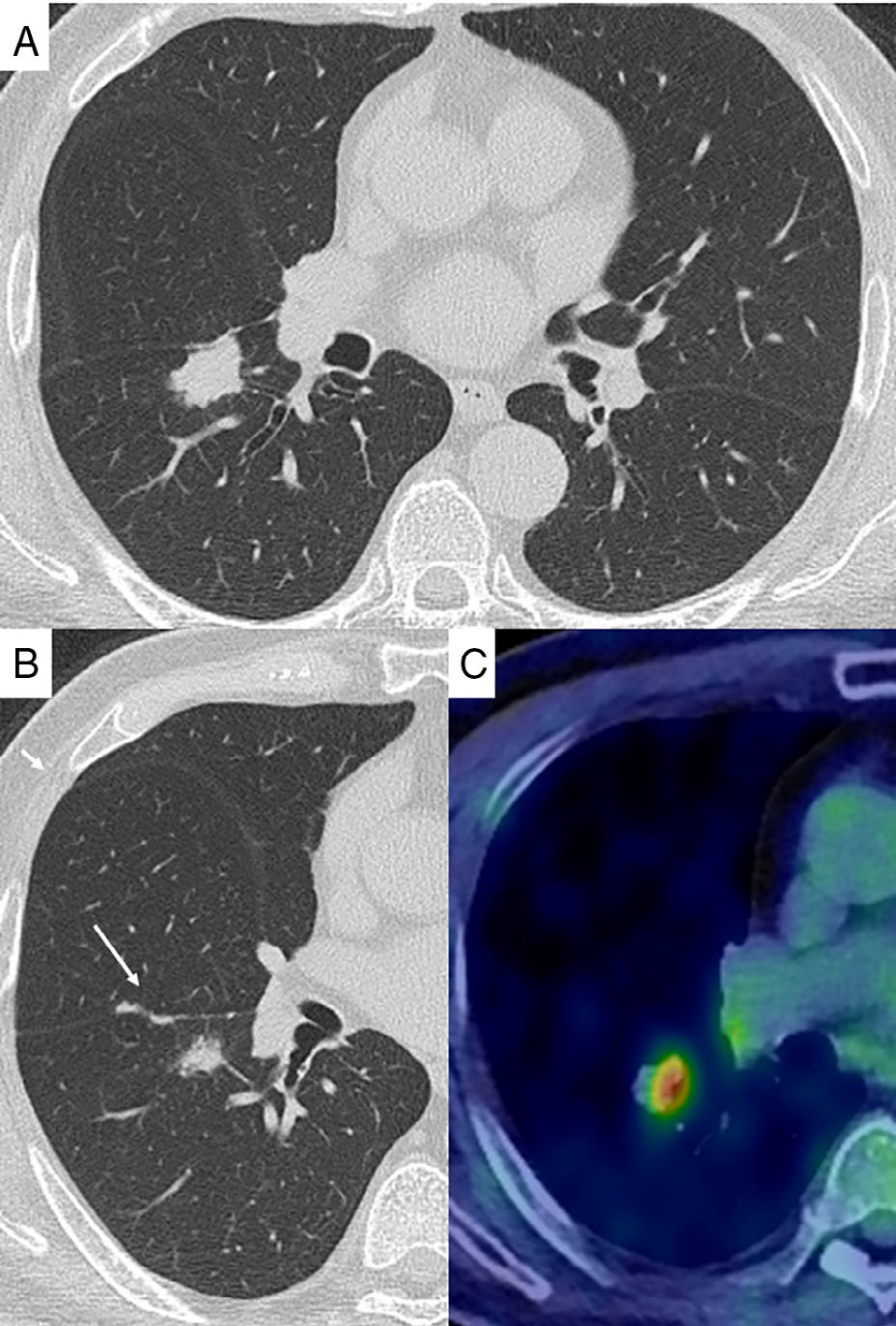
(A, B) Chest computed tomography (CT) scan showed a lung nodule measuring 26 × 14 mm with multiple tiny nodules on the pleural surface (arrow). (C) ^18^F‐fluorodeoxyglucose‐positron emission tomography scan showed a maximum standardized uptake value of 4.69 within the nodule.
